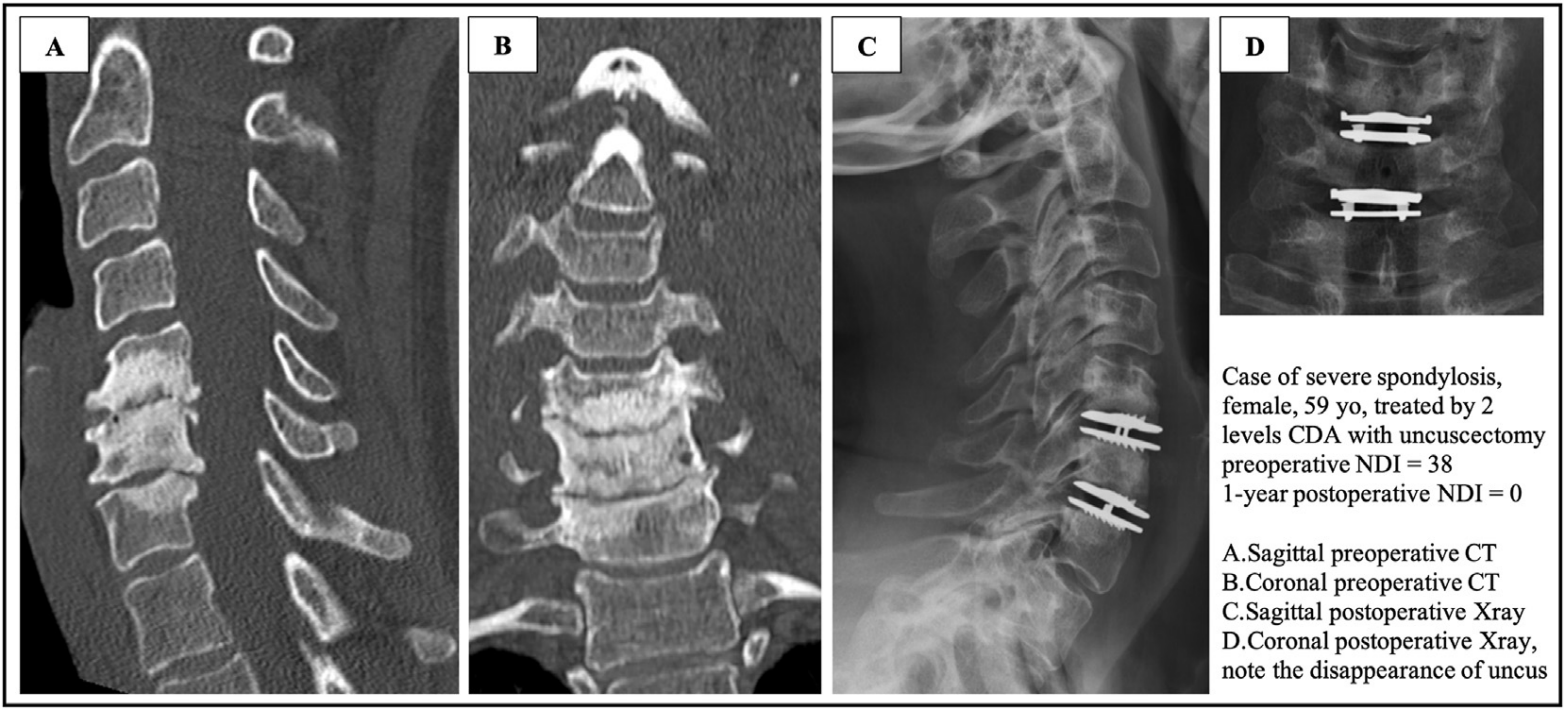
Case of severe spondylosis, female, 59 yo, treated by 2 levels CDA with uncuscectomy; preoperative NDI = 38; 1-year postoperative NDI ​= ​0; A. Sagittal preoperative CT B. Coronal preoperative CT C. Sagittal postoperative Xray D. Coronal postoperative Xray, note the disappearance of uncus.
